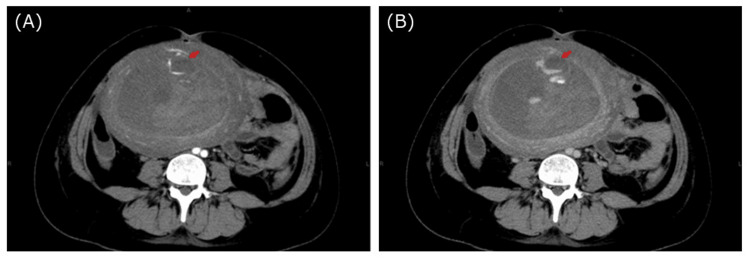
Abdominal contrast-enhanced CT. (A-B): The arrow indicates a hematoma with extravasation.
